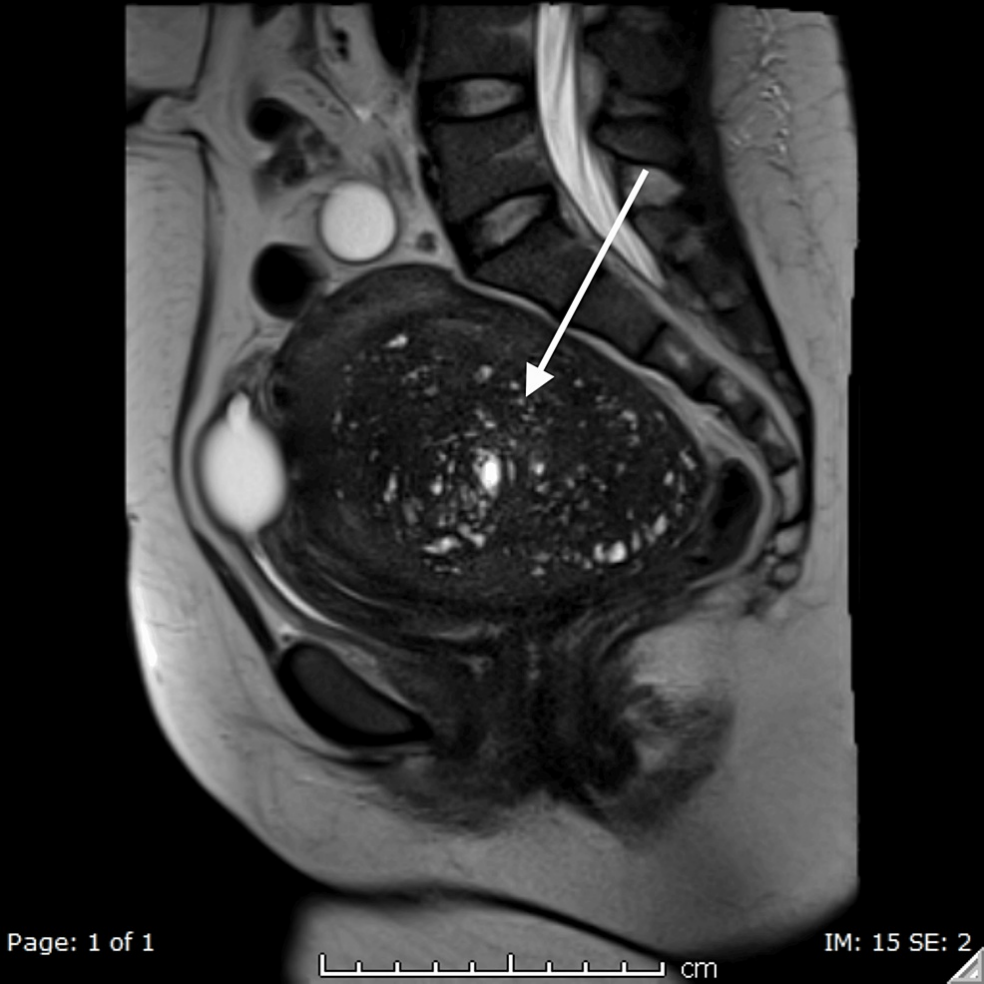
Sagittal T2-weighted images showing adenomyosis with glandular, cystic, and solid components
